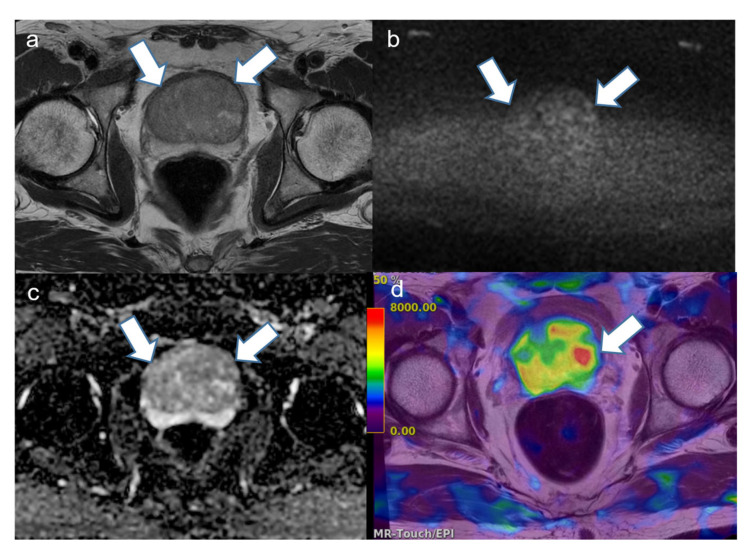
A representative case of a 73-year-old man with a benign prostatic hyperplasia (BPH) nodule (arrows) showing the internal heterogeneity of the stiffness value. (a) T2-weighted image shows a large BPH nodule with heterogeneous signal intensity (SI) in the transition zone. (b) Diffusion-weighted image (b value, 2000 s/mm^2^) showing a heterogeneous high SI in the BPH nodule. (c) The corresponding apparent diffusion coefficient map also shows a reciprocal heterogeneous low SI in the BPH nodule. (d) Elastogram fused with T2-weighted image shows a wide range of stiffness values in the BPH nodule. Some portions indicate a stiffness value of 8 kilopascals, which is similar to that of prostate cancer.
